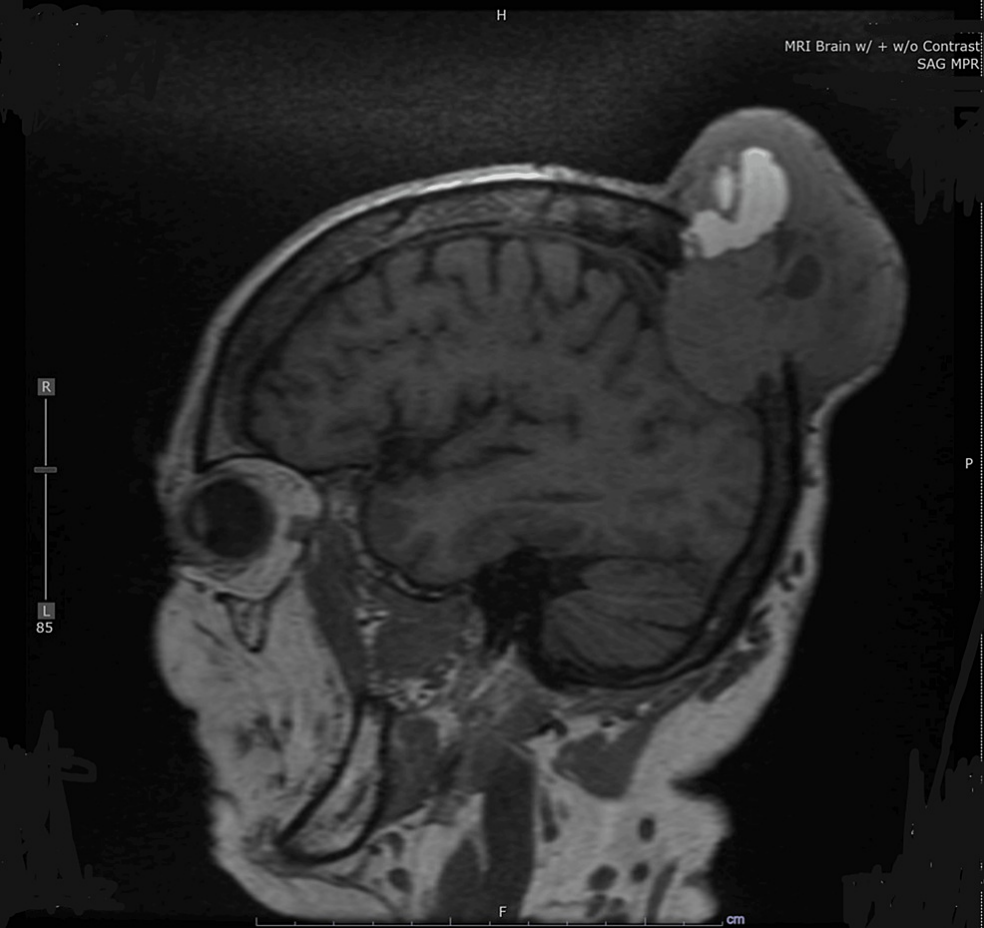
Sagittal MRI of the head with and without contrast. MRI of the brain depicting a large 8.9 cm x 9.2 cm x 7.4 cm solid-cystic mass in the posterior parietal calvarium with bony destruction and both extracranial and intracranial extensions. MRI, magnetic resonance imaging
